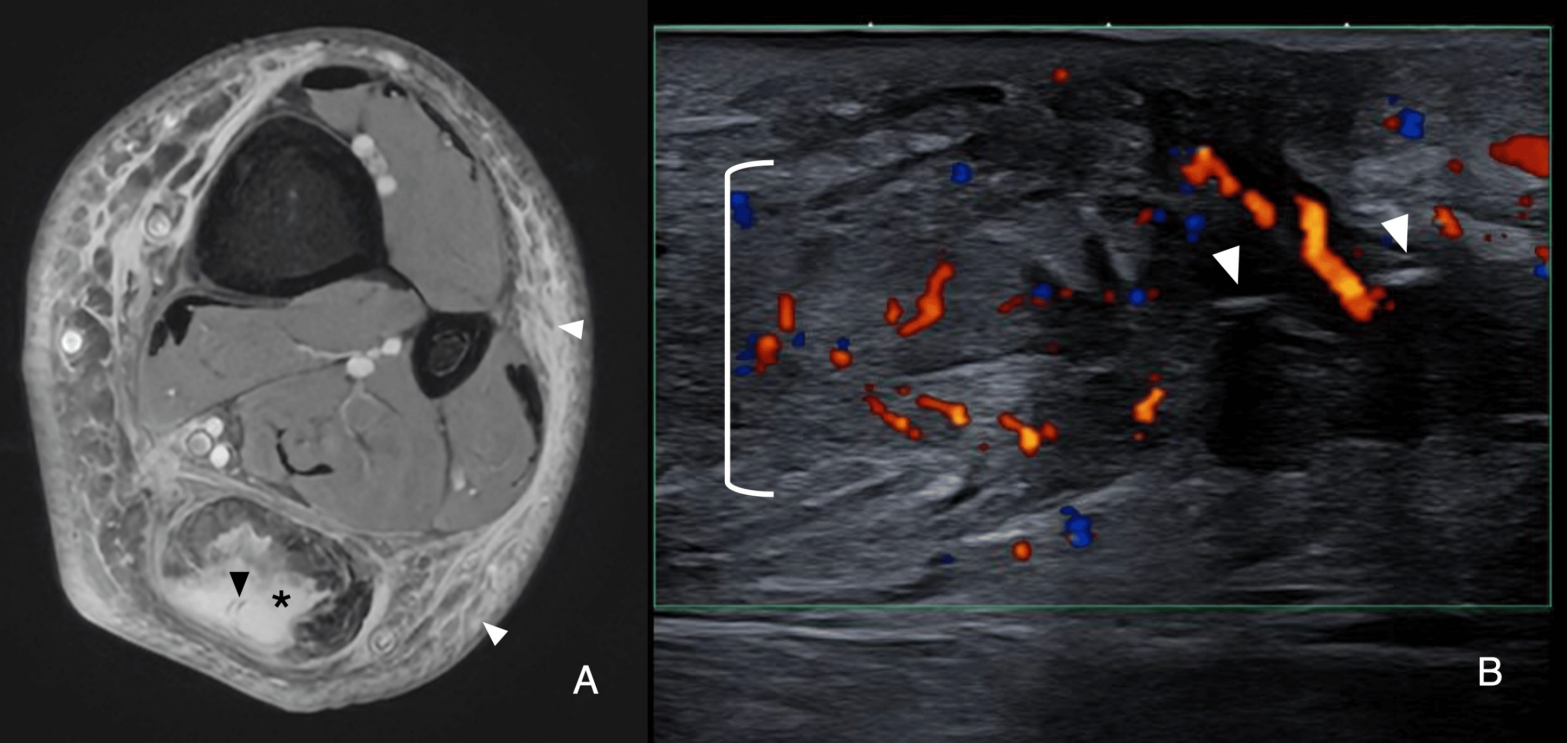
MRI and ultrasound (Patient 2) A) Axial PDFS reveals a cystic-like lesion (black asterisk) surrounding the sutures (black arrowhead), with associated soft tissue edema (white arrowheads). B) Long-axis color Doppler shows Doppler hyperemia (white bracket) surrounding the sutures (white arrowheads). PDFS, proton density fat-saturated
